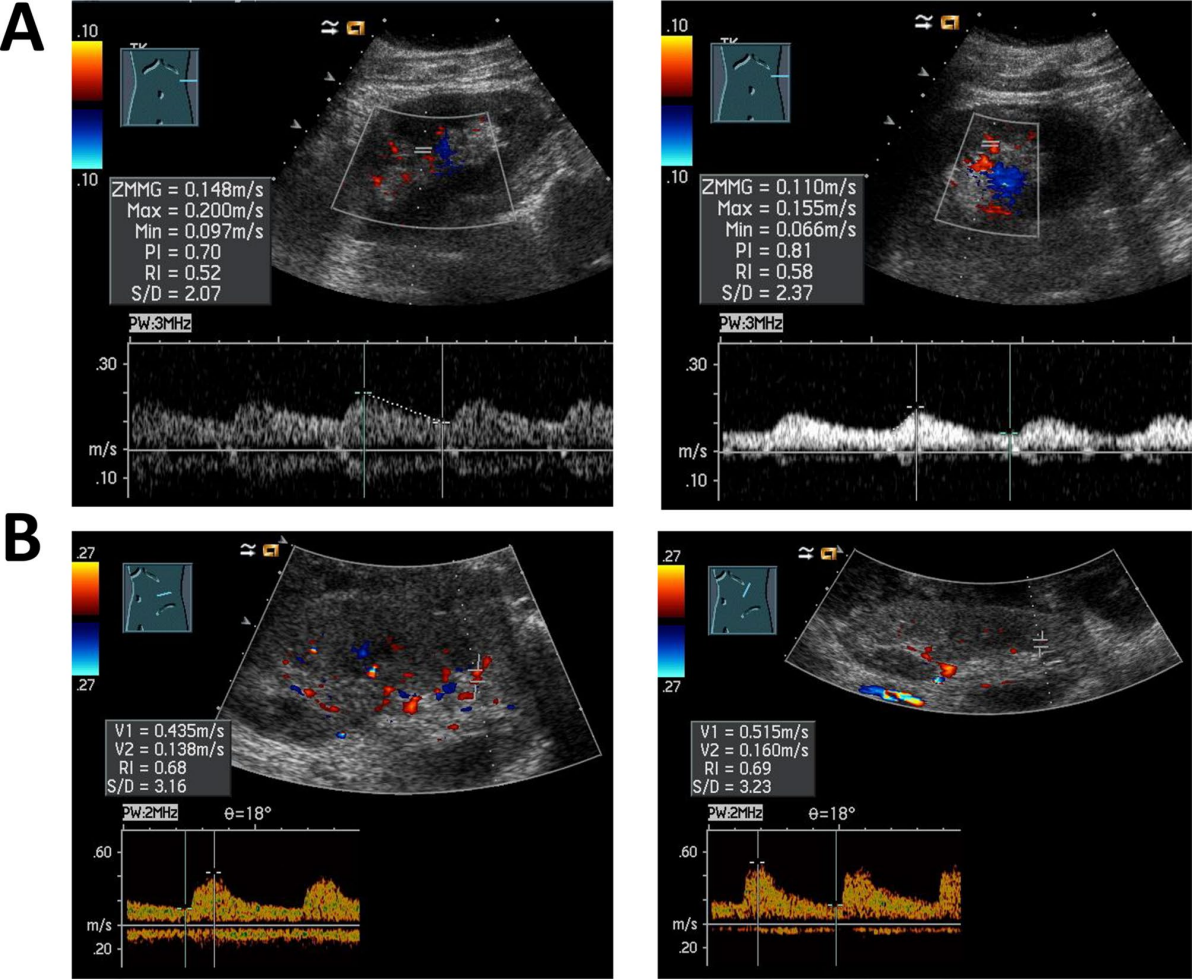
Renal doppler ultrasound before and after treatment for acute left aorta-renal bypass occlusion. A – both images represent the renal doppler ultrasound taken during the patient’s presentation. The left renal artery was not identifiable; however, a post-stenotic waveform was detected in renal parenchyma. Resistive indices (RI) ranged from 0.52 to 0.58, which is below that is expected for the 40-year-old male with a history of arterial hypertension. Additionally, prolonged acceleration time in Doppler waveforms was identified. B – after the graft placement between the aorta and the renal artery, doppler ultrasound showed an improved perfusion profile of the left kidney. RI´s exhibited a systolic peak, ranging from 0.64 to 0.66. Moreover, blood flow in the renal artery was measured with a maximal value of 195 cm/s
